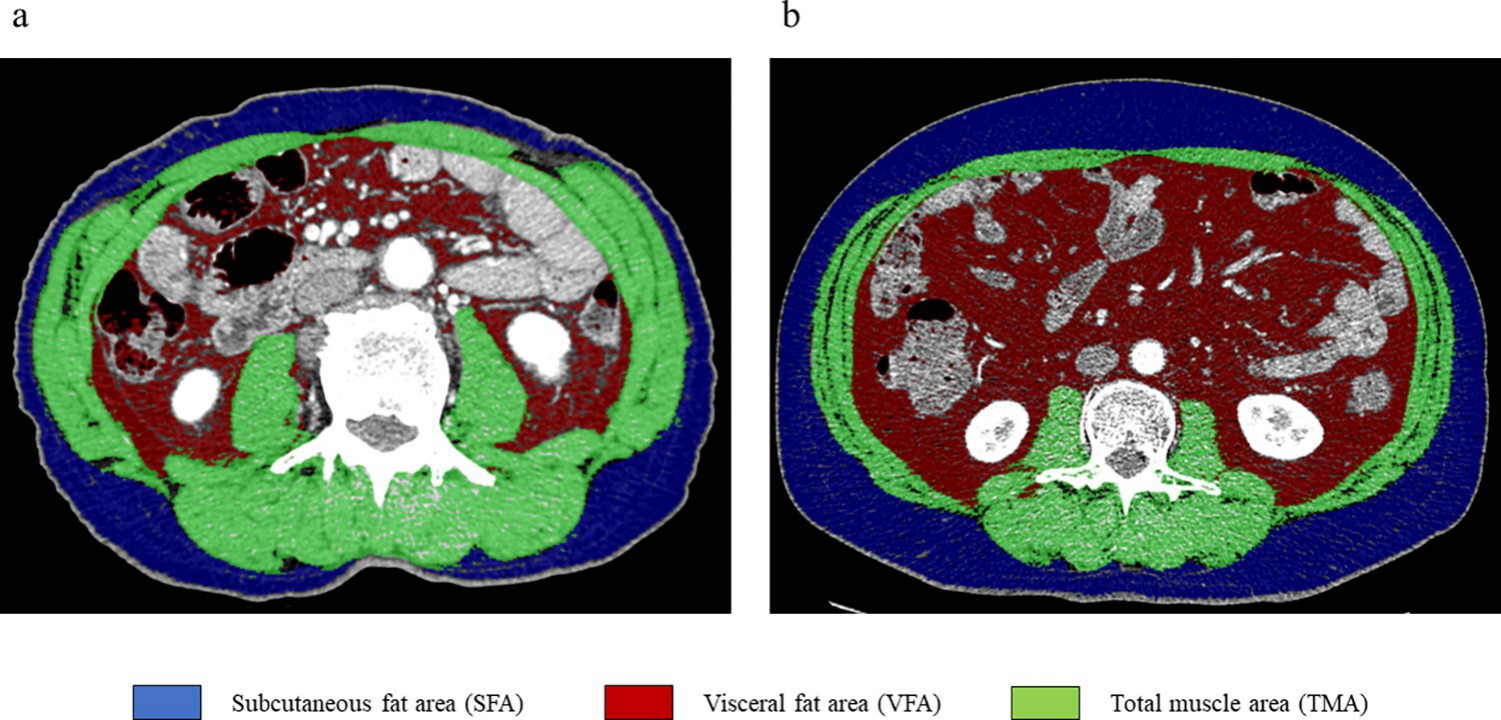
Computed tomography image analysis of a third lumbar vertebra. Green, muscle area; red, visceral fat area; blue, subcutaneous fat area. (a) A patient without sarcopenic obesity. (b) A patient with sarcopenic obesity.
